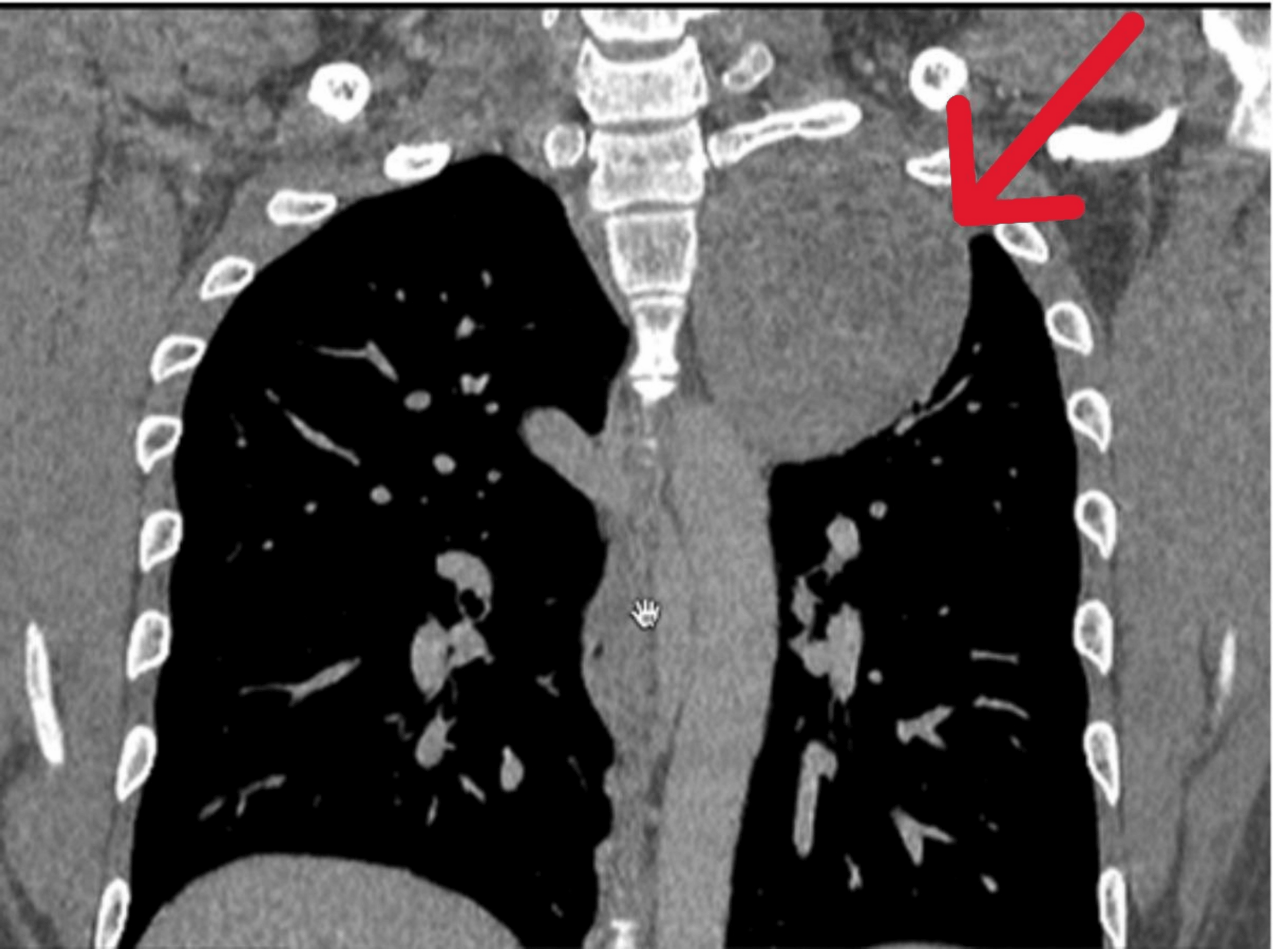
Coronal view of contrast-enhanced computed tomography (CECT) of thorax. The arrow shows the thoracic mass over the left upper region (coronal view).
